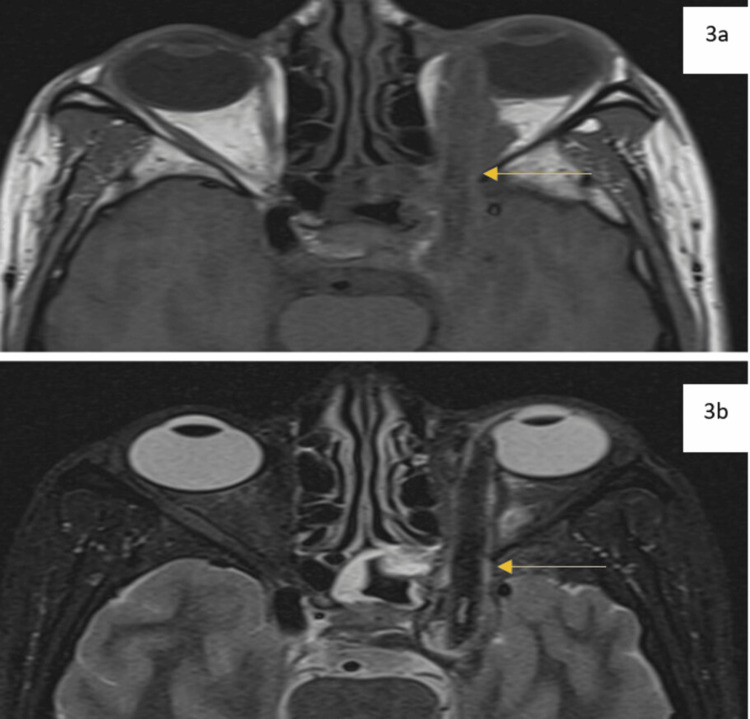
MRI images. a: T1 weighted magnetic resonance imaging reveals a wooden foreign body as a linear hypointense structure on the left side. (yellow arrow). b: T2 weighted magnetic resonance imaging reveals a hypointense foreign body with optic nerve compression at the orbital apex from granulation tissue (yellow arrow).
